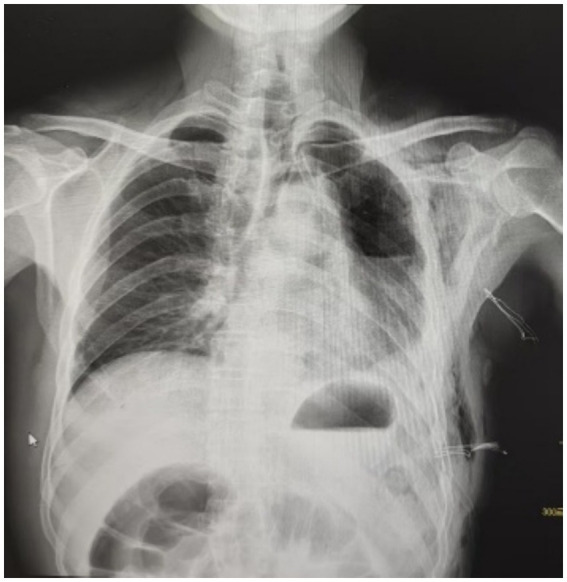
The patient’s second hospitalization chest radiograph revealed 40% compression of the left lung with moderate pneumothorax and pleural effusion.
